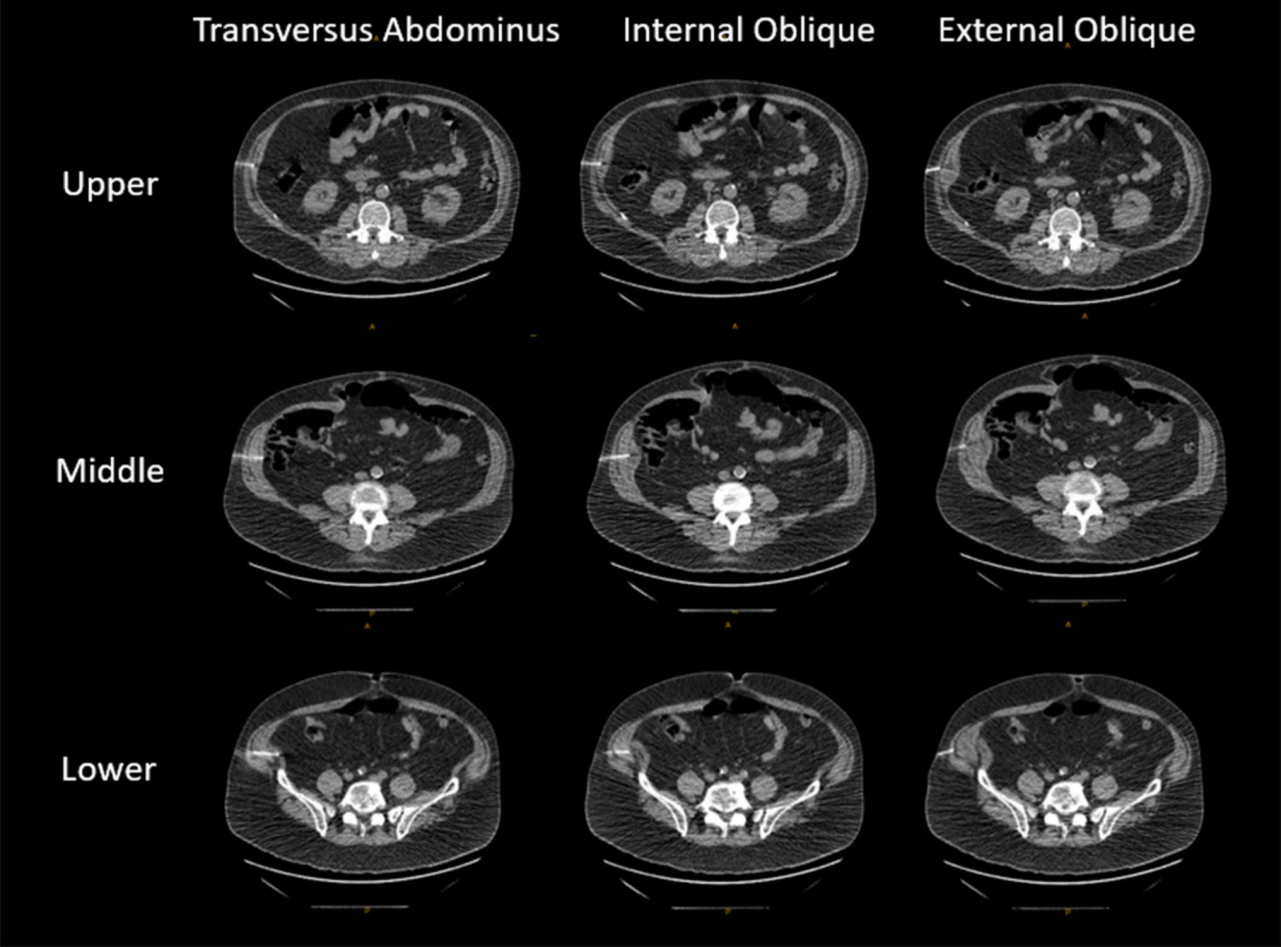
Computed tomography (CT)-guided injection of OnabotulinumtoxinA into the abdominal wall muscles, with three injection points (upper, mid, and lower) per muscle, totaling nine injections per side. The injections on the right side are shown here
